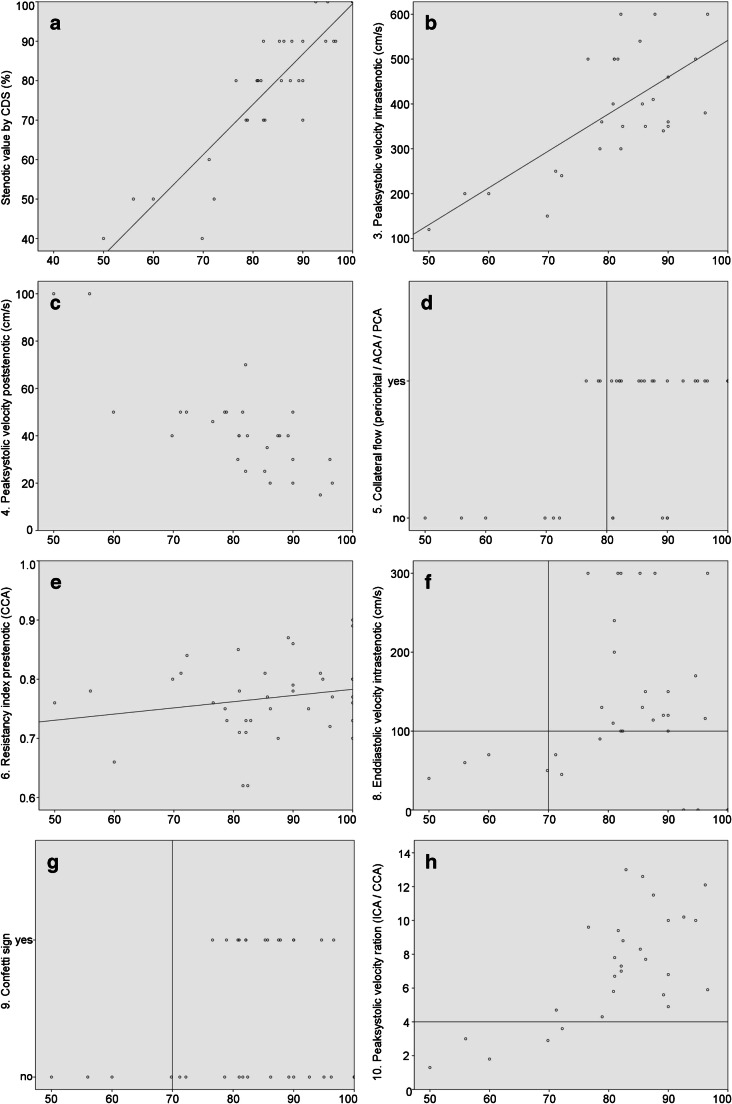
Correlation of the sonographic stenotic value and selected ultrasound criteria of the 2nd sonographer (y-axis) with the stenotic value assessed by digital subtraction angiography by the 2nd neuroradiologist (x-axis, in %). Overall, there was a good correlation between DSA and velocity-based criteria (b, c, f, h). Both criteria that were dichotomised into present or absent (collateral flow (d) and confetti sign (g)) confirmed high-grade stenosis when positive but could not rule out a relevant stenosis when negative. The vertical and horizontal bars in d, f, g, h indicate the boundaries that are proposed to discriminate between a moderate and severe stenosis [9, 11]
